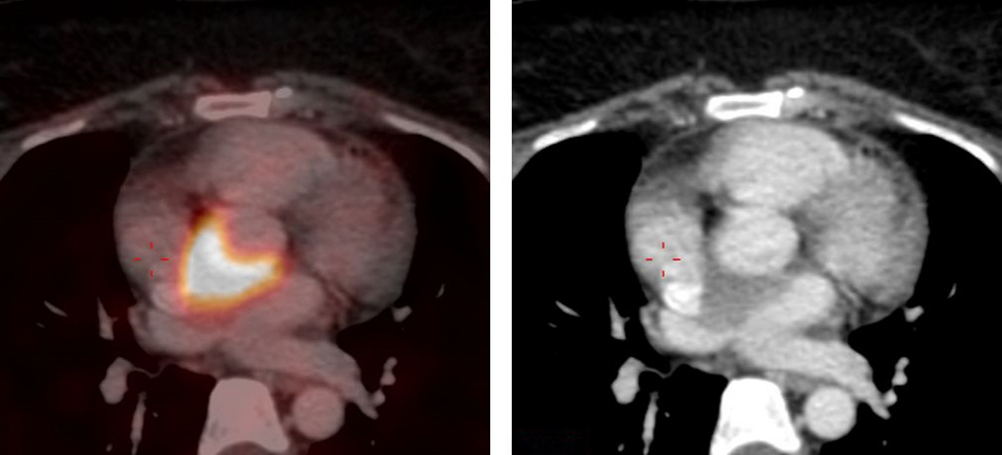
Left: ^18^F-fludeoxyglucose uptake in atrioventricular groove. Right: CT scan showing deposit in the atrioventricular groove.
